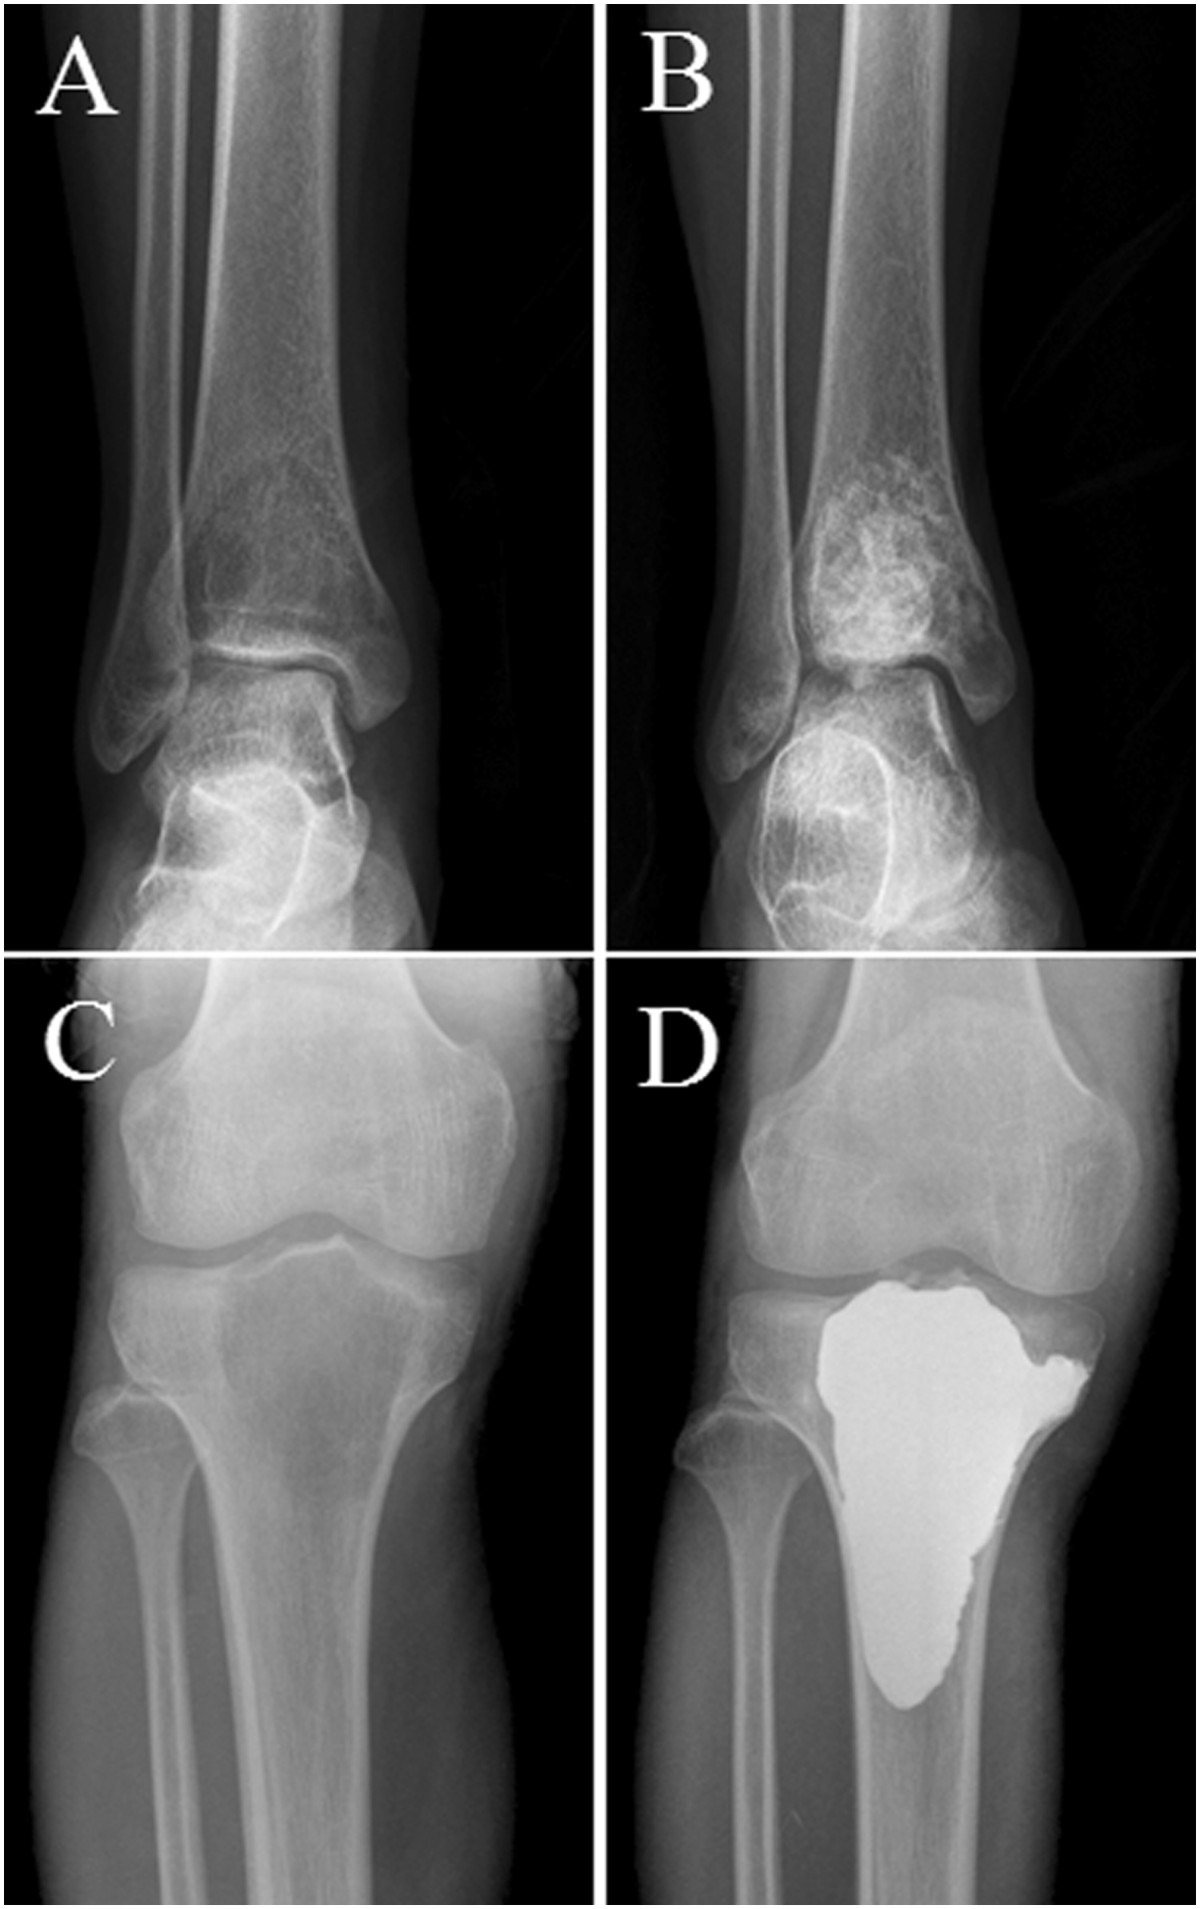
Typical radiograph of GCT of the long bone. Anteroposterior radiograph shows a lytic lesion in the distal tibia (A) and proximal tibia (C). Anteroposterior radiograph showing the results after agreessive curettage and filling the bone defect with bone grafts (B) and bone cement (D).
